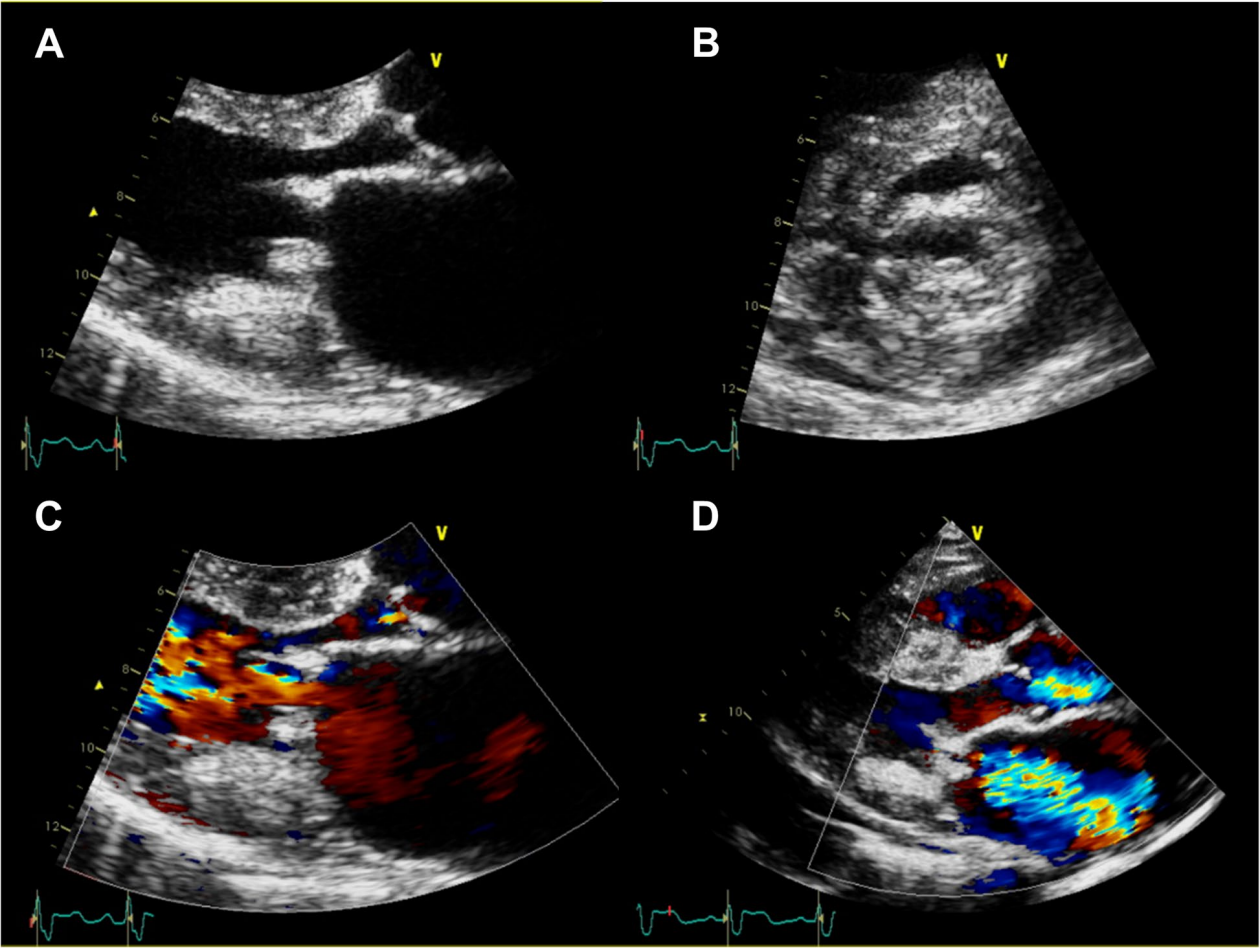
Parasternal view on transthoracic echocardiogram. A Zoomed view of parasternal long axis view, demonstrating thickened mitral valve leaflets. B Parasternal short axis view demonstrating thickened mitral valve leaflets and restricted valve opening. C Accelerated mitral inflow during diastole in the color Doppler, indicating severe mitral stenosis. D Concomitant mitral regurgitation observed in the color Doppler
